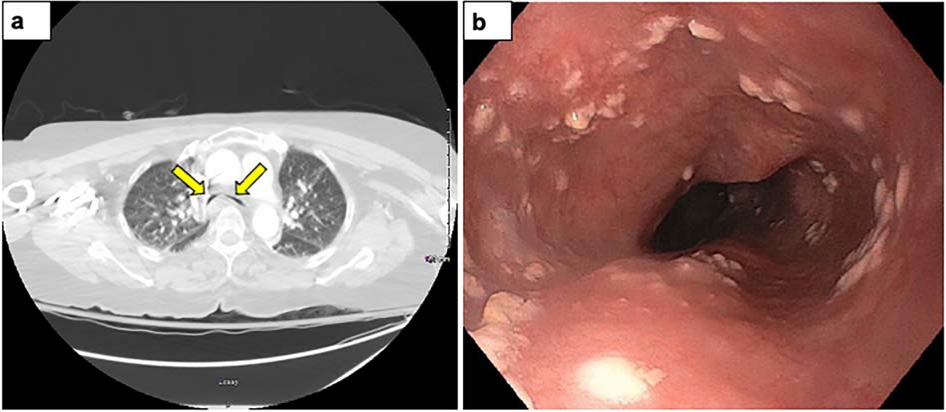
(a) Transverse computed tomography image showing severe tracheobronchomalacia with collapsing right and left main bronchi (arrows). (b) Bronchoscopic image of the trachea with decreased anteroposterior diameter showing evidence of tracheobronchomalacia.
